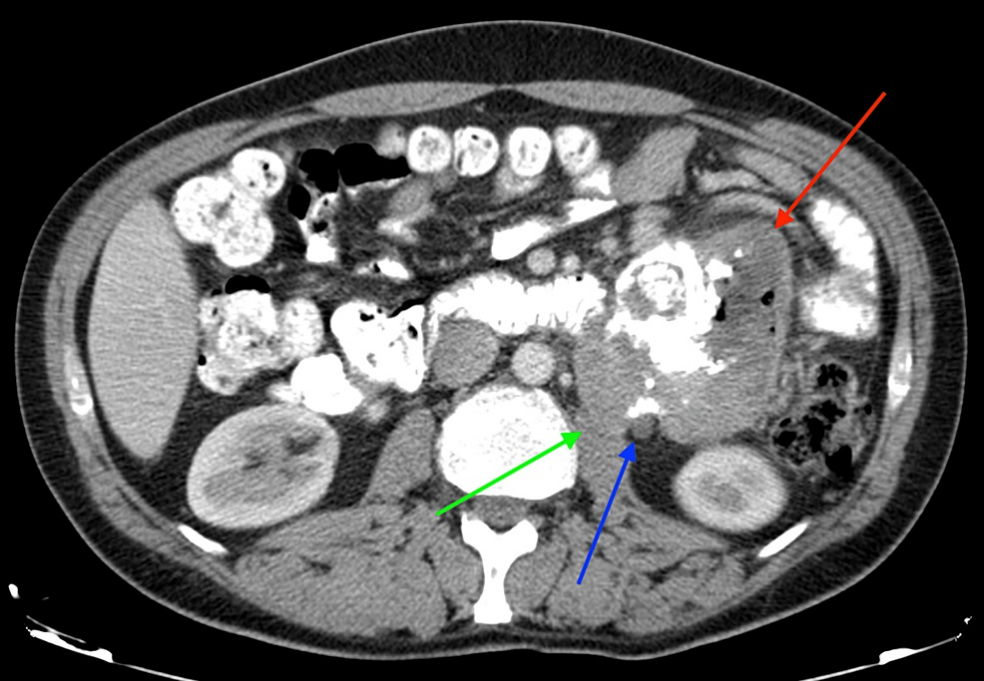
Axial CT appearance of the retroperitoneal mass The red arrow shows the lesion, which displays central foci of calcification, air, and areas of necrosis. The green arrow shows the left psoas muscle infiltration, and the blue arrow shows the strangulation of the left ureter by the lesion in the retroperitoneal space CT: computed tomography
